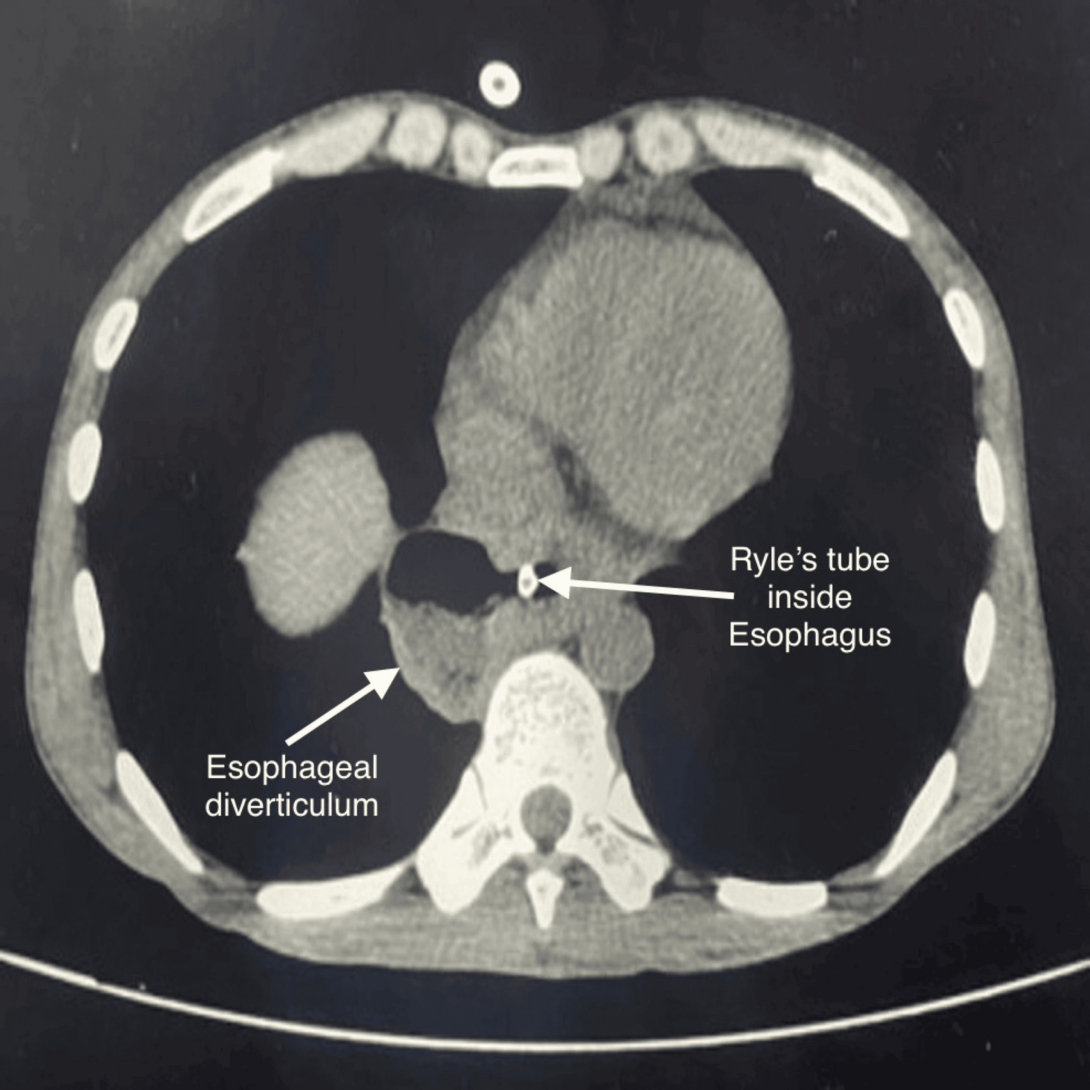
Preoperative chest computed tomography scan showing dilatation of the esophagus and an epiphrenic diverticulum measuring 4.19 x 2.93 cm.
